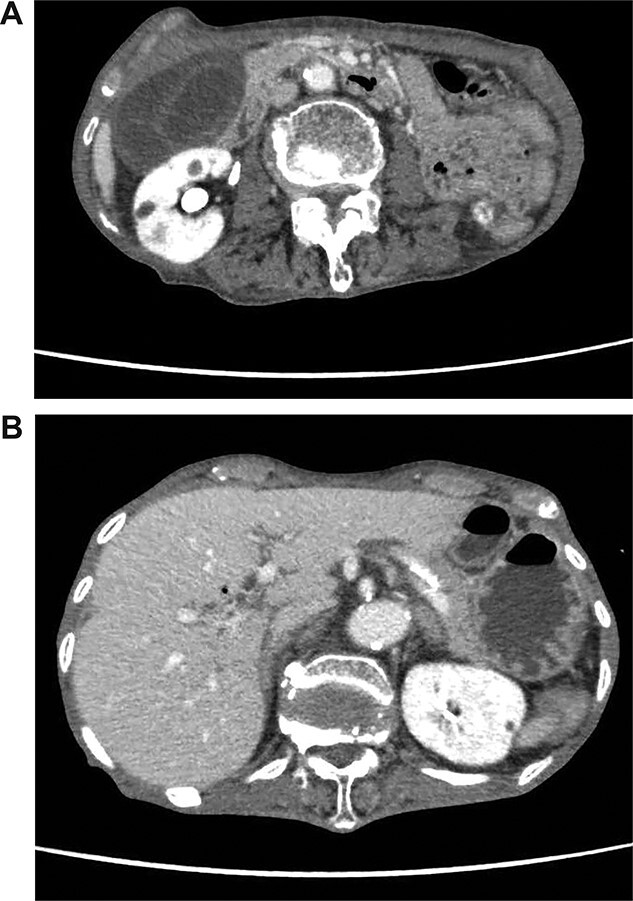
(A) Abdominal CT shows a poorly enhanced and thickened wall of the gallbladder. (B) Abdominal CT shows tiny bubbles in the intrahepatic portal venous branches.
